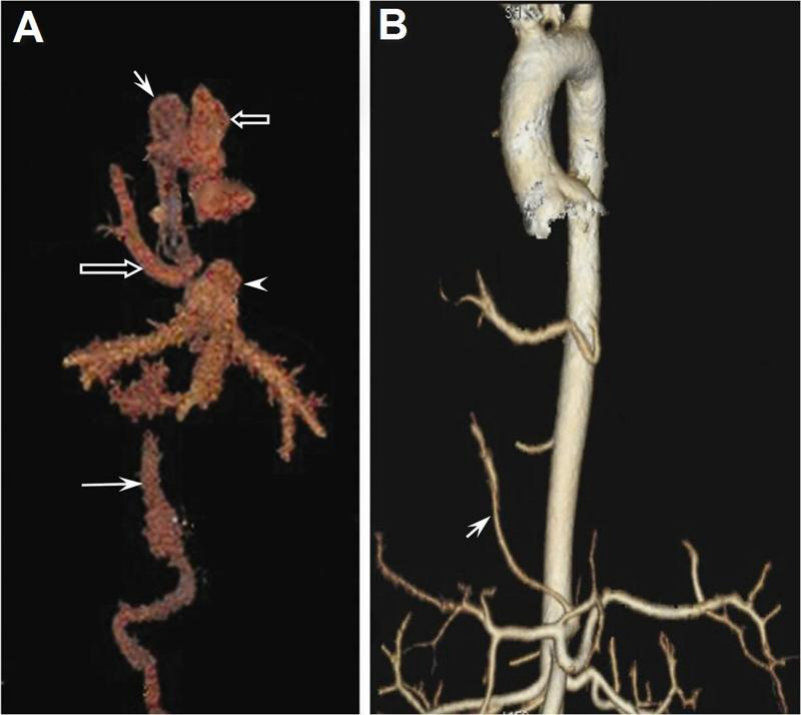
A - Frontal projection of the venous phase of 3D CT angiography with volume rendering, which is obtained after the operation. Annotated structures are: short solid arrow = enlarged azygos vein; long solid arrow = IVC, which is interrupted at the hepatic level; short hollow arrow = SVC; long hollow arrow = Redirected anomalous pulmonary vein; arrow head = hepatic vein, which drains into right atrium. B - The arterial phase depicts the anomalous systemic artery (arrow) arising from the celiac trunk and intending to supply the base of the right lung, which is ligated at the level of the diaphragm.
